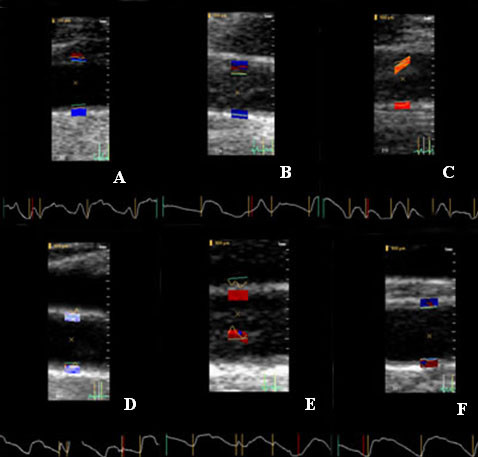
Limitations – artifacts. Artifacts with carotid tissue Doppler caused by technical issues: A – artifact from breathing which caused the artery to move in and out of the image plane; B – Excessive noise in the lumen which the analysis program picked up as tissue; C – Reverberation causing inaccurate detection of the vessel wall; D – Excessive movement of the artery during the cardiac cycle; E – Reverberation causing inaccurate detection of the vessel wall; F – Very little movement detected in the artery at peak pulse pressure and flow resulting in poor quality waveforms.
